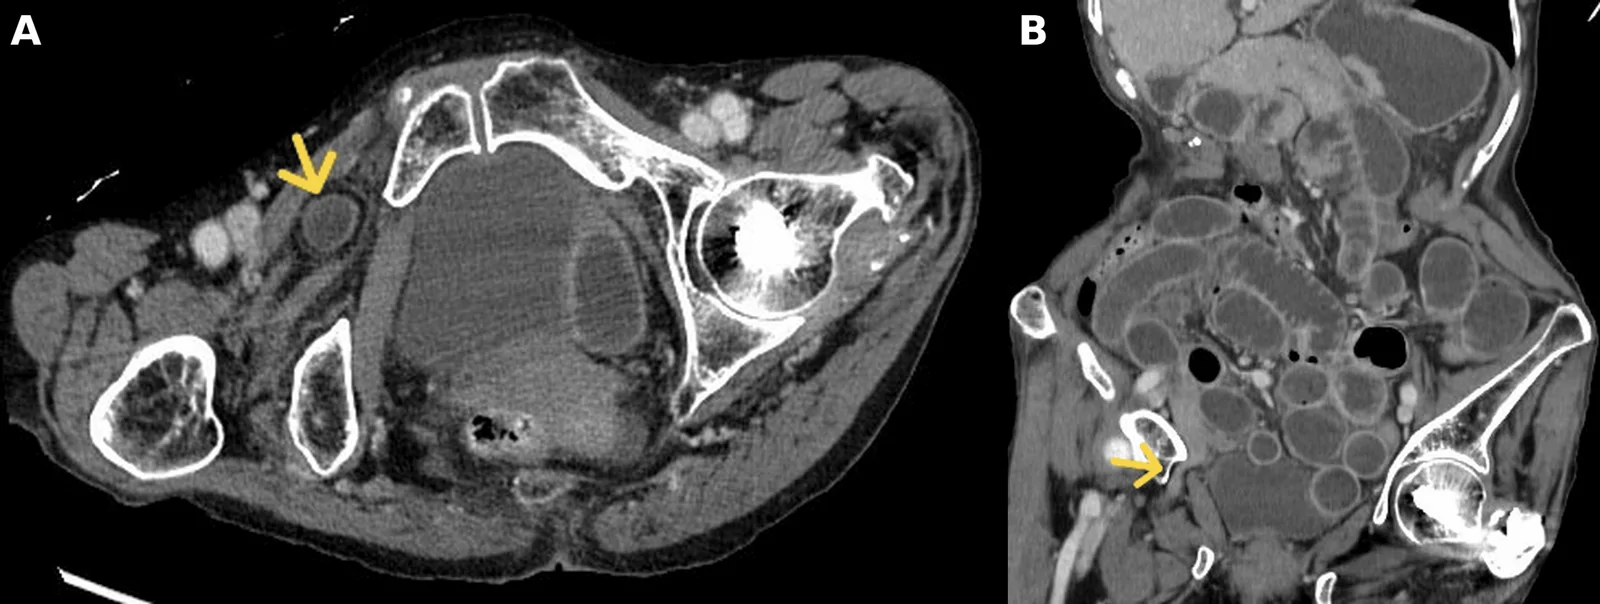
Contrast-enhanced abdominal computed tomography (CT) of the pelvis Axial (A) and coronal (B) contrast-enhanced CT images of the pelvis showing a small bowel incarceration within the right obturator foramen (yellow arrows), consistent with a right obturator hernia.
